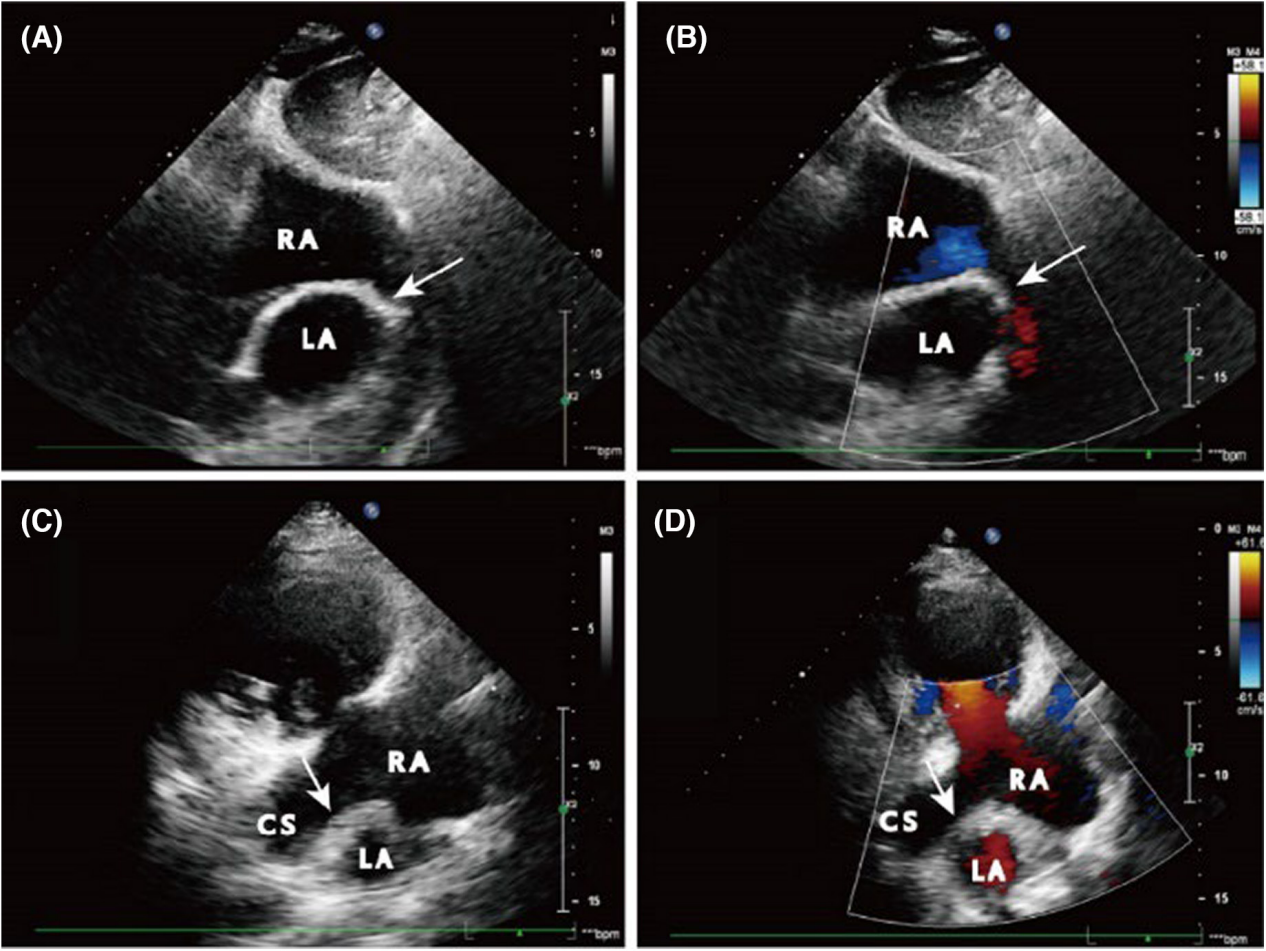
Two‐dimensional echocardiography at 1 week postoperatively. (A, B). Subxiphoid two‐atrial view: (A). two‐dimensional ultrasound shows no significant interruption between the LA and CS as well as the atrial septum (shown by white arrows); (B). Color Doppler shows no color shunt signal between the LA and CS (shown by white arrows); (C, D). Long‐axis view of the left ventricle: (C). Two‐dimensional ultrasound shows an obvious interval echo between LA and CS (shown by the white arrow); (D). Color Doppler shows that there is no blood flow signal connecting the two (shown by white arrows). (LA, left atrium; RA, right atrium; RV, right ventricle; CS, coronary venous sinus).
